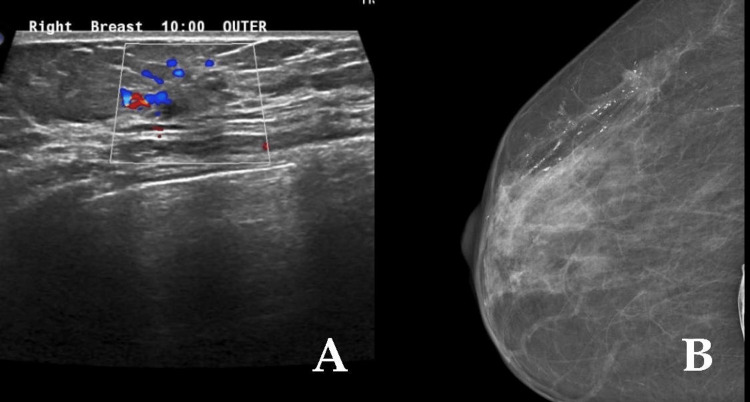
Case 1 - ultrasound and mammography. (A) Right breast US and (B) right breast mammography revealed that the right breast was BIRADS 4C. BIRADS: breast imaging-reporting and data system
